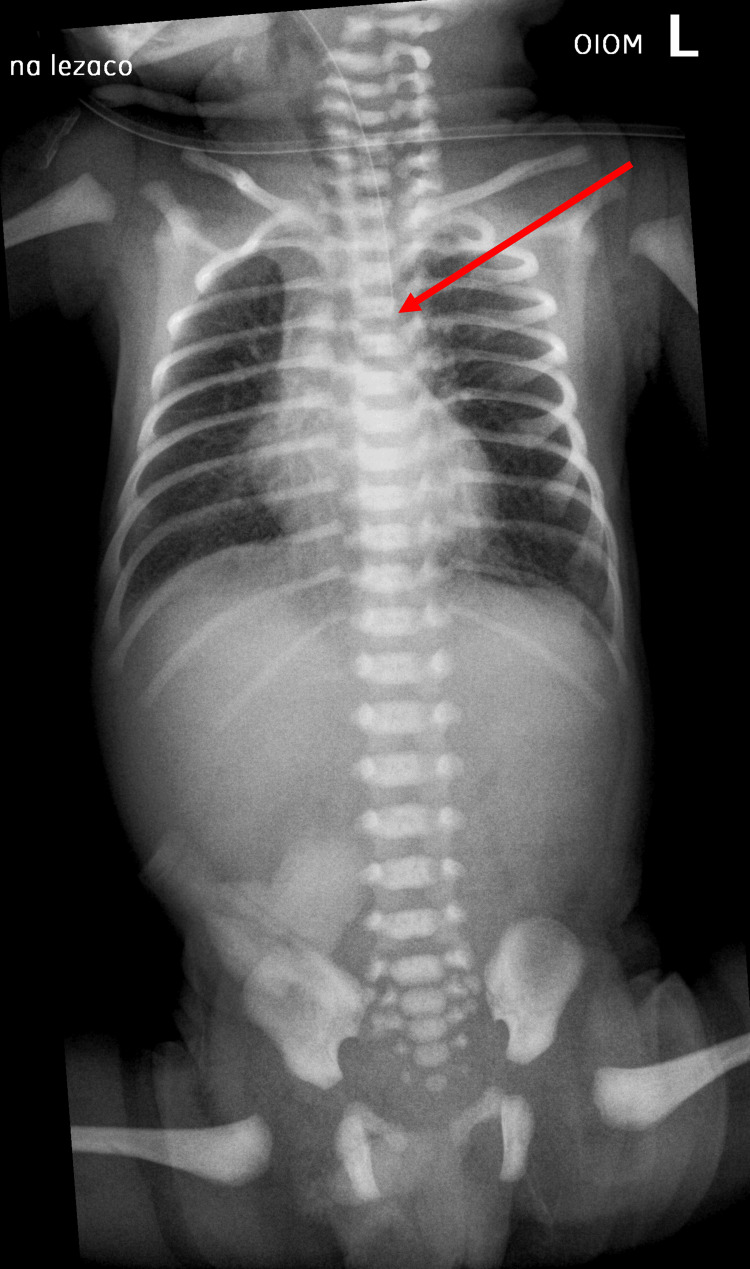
The "babygram" X-ray reveals a gasless stomach and intestines. An orogastric tube is inserted. The red arrow points to the end of the orogastric tube, which could not be passed further down.
